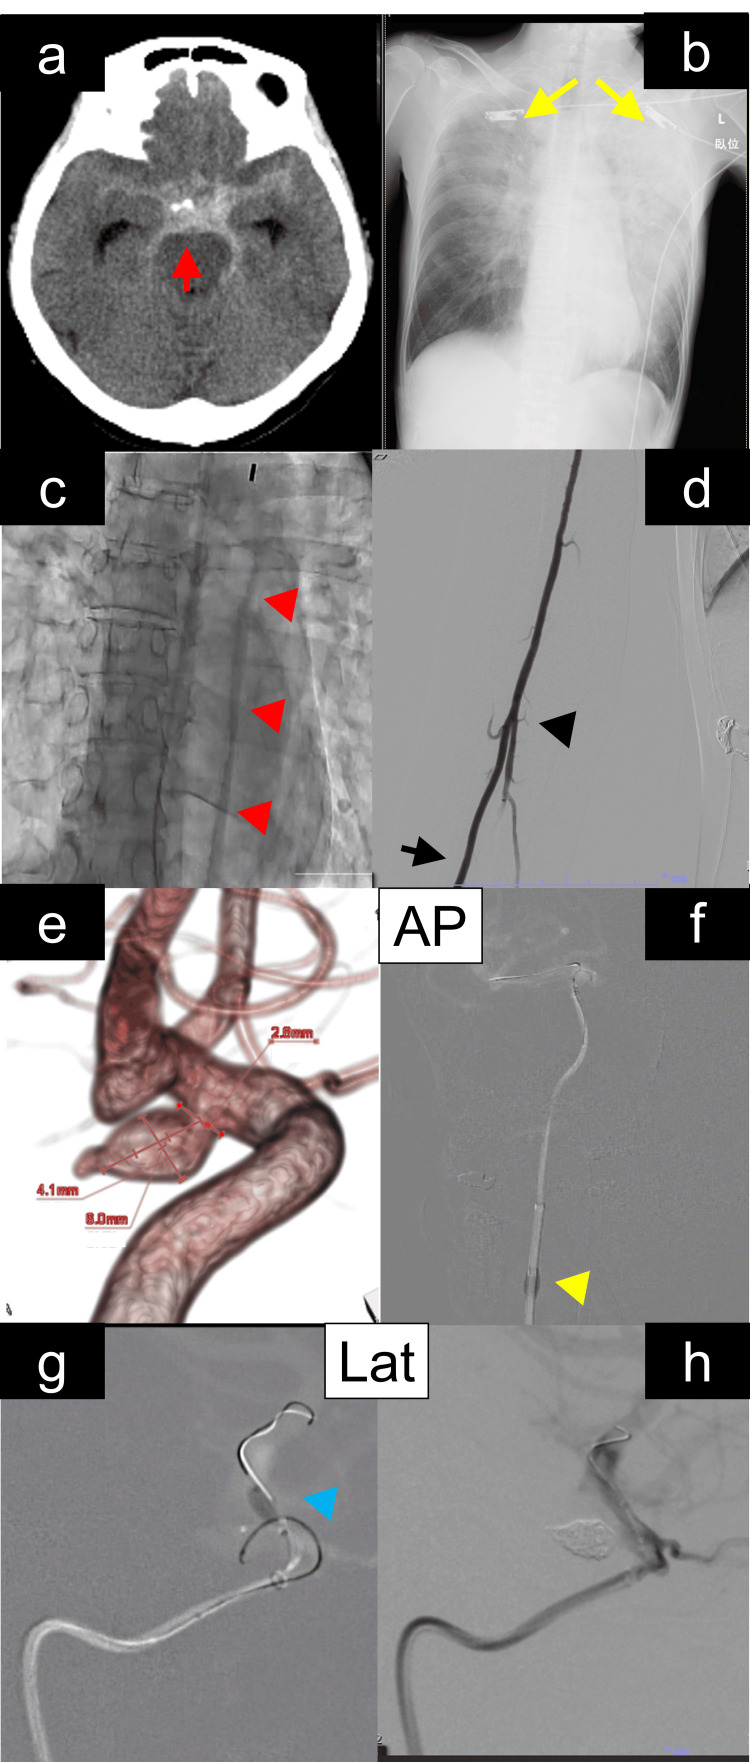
Radiological and procedural findings in a patient with subarachnoid hemorrhage, Takotsubo cardiomyopathy, and aneurysmal embolization under intra-aortic balloon pump (IABP) support a) Initial non-contrast head computed tomography revealed a subarachnoid hemorrhage predominantly in the basal cisterns (red arrow). b) Chest radiography showed signs consistent with Takotsubo cardiomyopathy and pulmonary edema (yellow arrow). c) IABP was placed via the right femoral artery, with the balloon positioned in the descending thoracic aorta (red arrowhead). d) Neuroendovascular intervention was performed via a distal radial artery approach while reviewing the vascular anatomy of the radial (black arrow), brachial artery, and the cubital portion (black arrowhead). e) Cerebral angiography demonstrated a right internal carotid-posterior communicating artery aneurysm with a bleb, measuring 8.0 mm in maximum diameter (excluding the bleb: 6.0 × 4.0 × 3.0 mm; neck: 2.8 mm). f) Proximal flow control was achieved using a balloon guiding catheter (yellow arrowhead). g) Distal flow control was established using a micro-balloon catheter (blue arrowhead). h) Complete flow arrest within the aneurysmal sac was confirmed with no contrast opacification observed. AP: anteroposterior, IABP: intra-aortic balloon pumping, Lat: lateral
